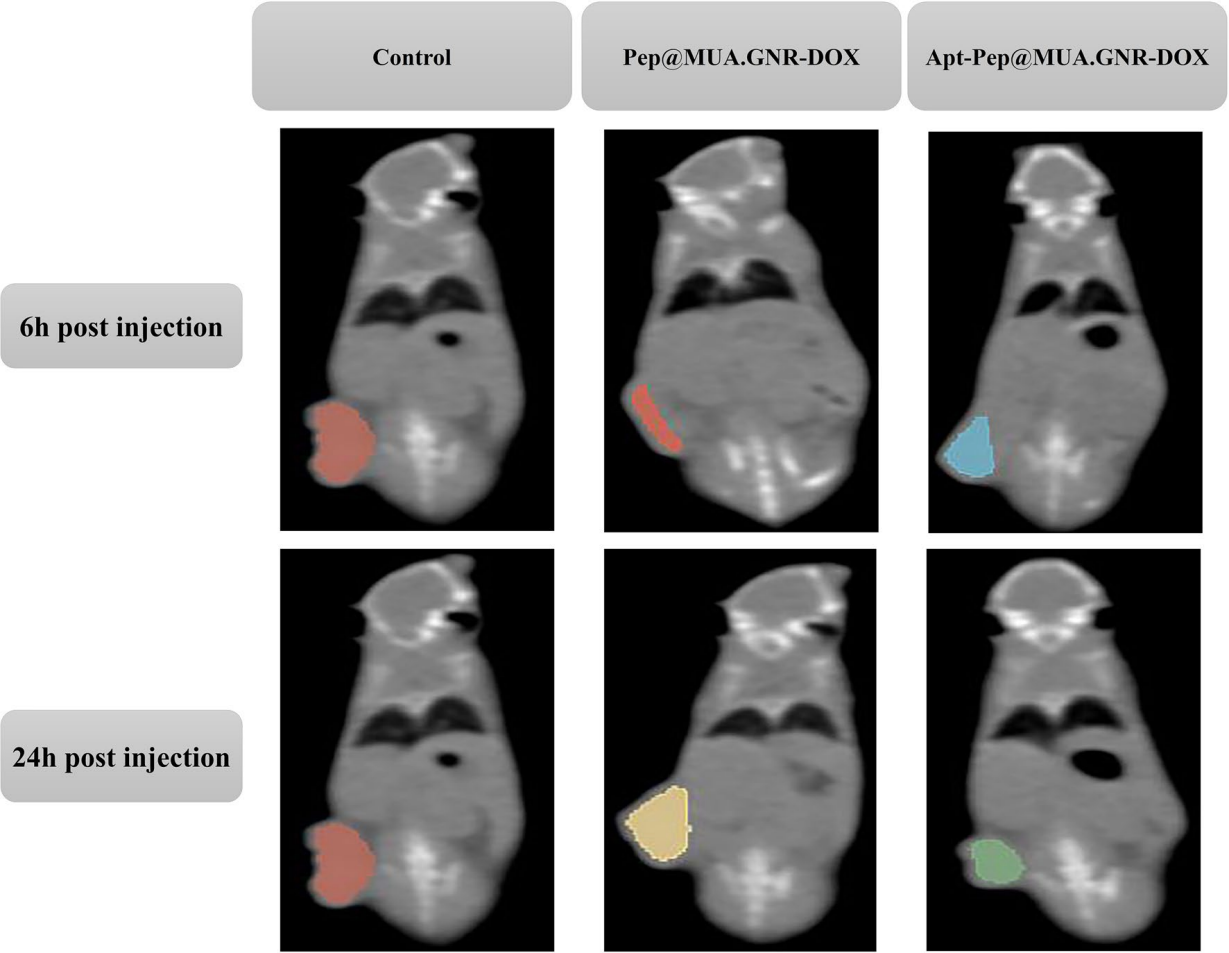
The in vivo CT scan coronal view of the tumor site 6 and 24 h post-injection of either Pep@MUA.GNR-DOX or Apt-Pep@MUA.GNR-DOX
